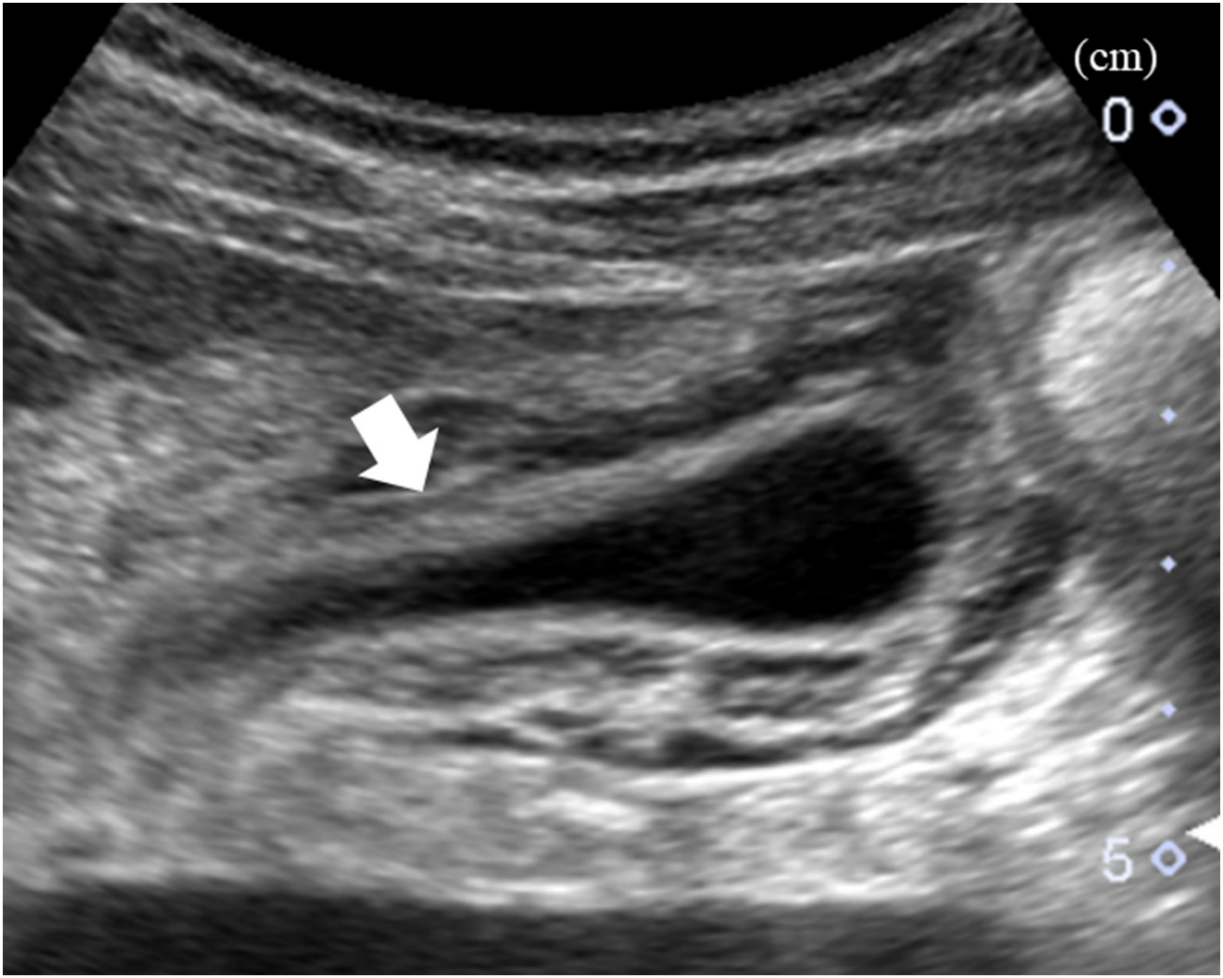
Findings of the gallbladder wall on abdominal ultrasonography on admission. Abdominal ultrasonography on admission shows the thickened gallbladder wall measuring 10 mm (arrow).
